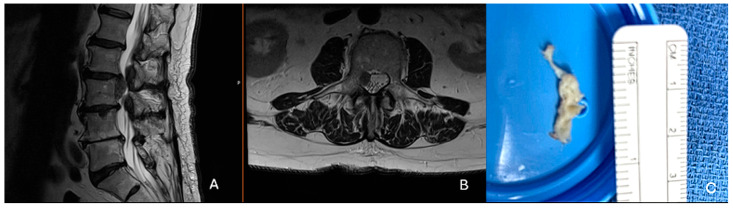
80-year-old male who is an ideal candidate for a right-sided L2–3 interlaminar endoscopic approach for a highly migrated disc herniation. (A): Preoperative T2-weighted sagittal MRI demonstrating the large, migrated disc herniation that has migrated to just rostral to the L3–4 disc space (B): Axial T2-weighted MRI at the mid L3 vertebrae (C): Picture of removed herniated disc fragment.
